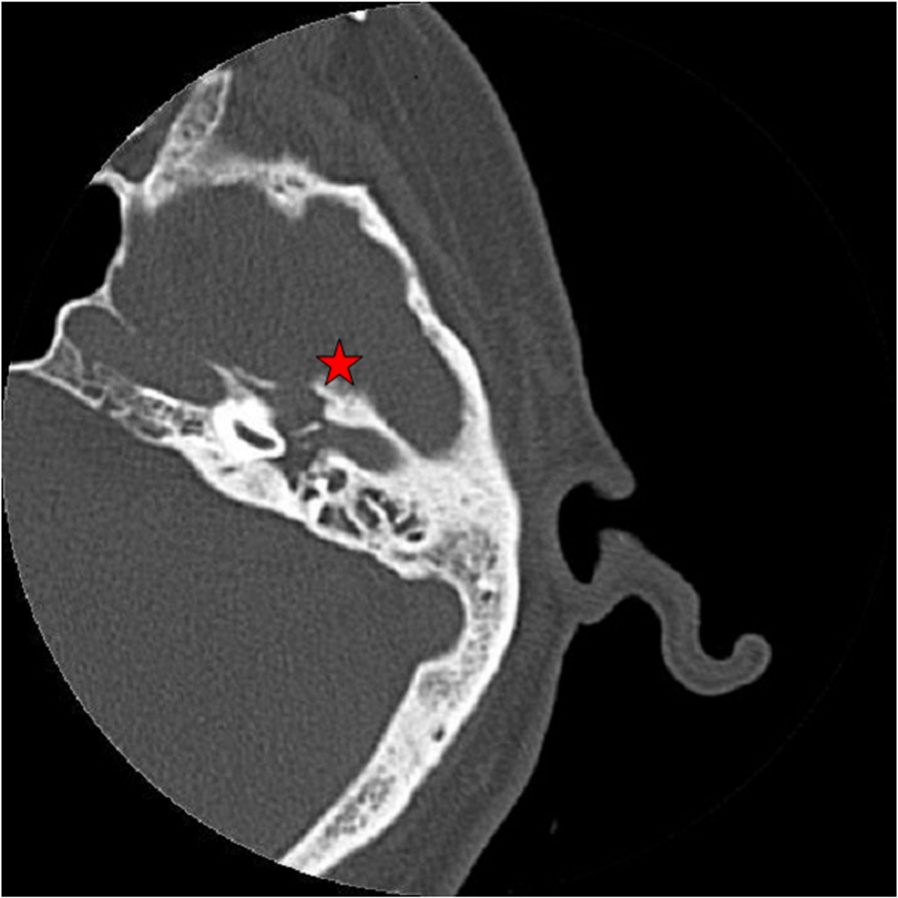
Petrous bone computed tomography scan shows occupation of the left middle ear and mastoid cells and erosion of the anterior wall of the middle ear (*star*)
